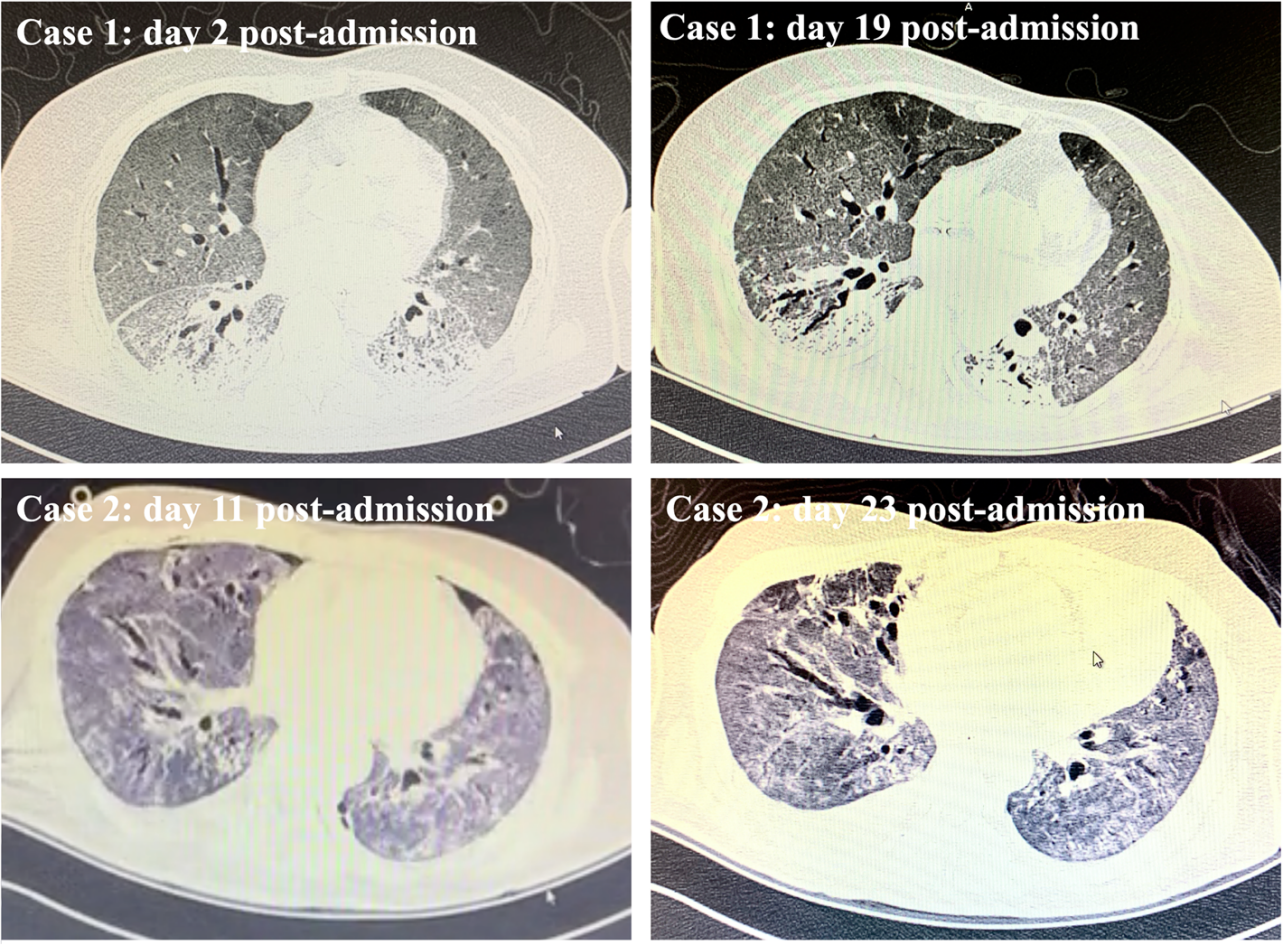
Left: transverse chest CT images from a 70-year-old female COVID-19 patient showing bilateral diffuse ground glass opacity, interstitial fibrosis, traction bronchiectasis and a small amount of lung consolidation in the dependent lungs on 2 and 19 days after invasive ventilation. Right: transverse chest CT images from a 42-year-old male COVID-19 patient showing bilateral ground glass opacity, interstitial fibrosis, left pleural effusion and traction bronchiectasis on 36 days and 48 days after invasive ventilation
